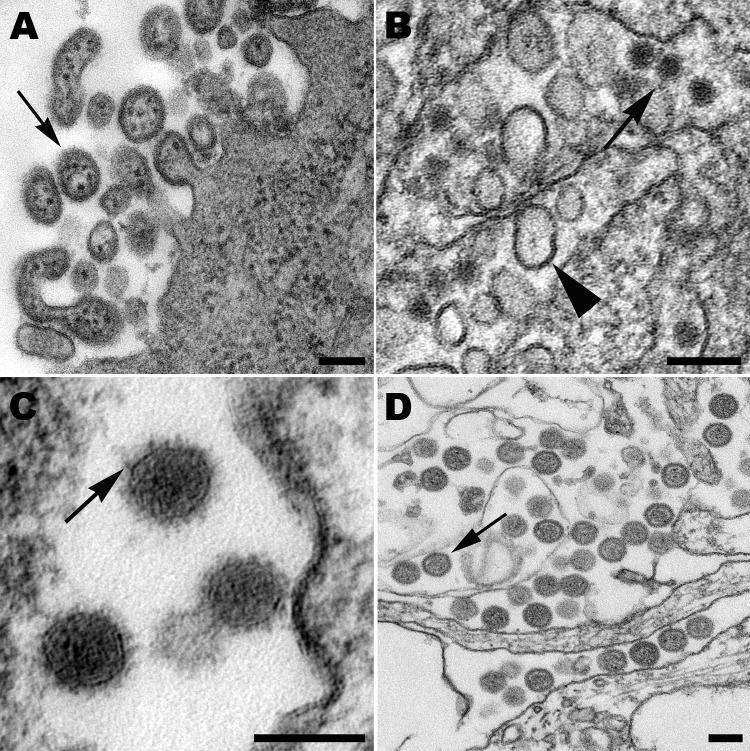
A) Noncontrast cranial computed tomographic (CT) scan of a 26-year-old immunocompetent man with influenza, showing diffuse cerebral edema (Ed) and bilateral parieto-occipital hematoma (H). B) Cranial CT scan with contrast injection, showing diffuse cerebral edema (Ed) and cord sign (arrow) related to a venous thrombosis (VT) of the superior sagittal sinus.
